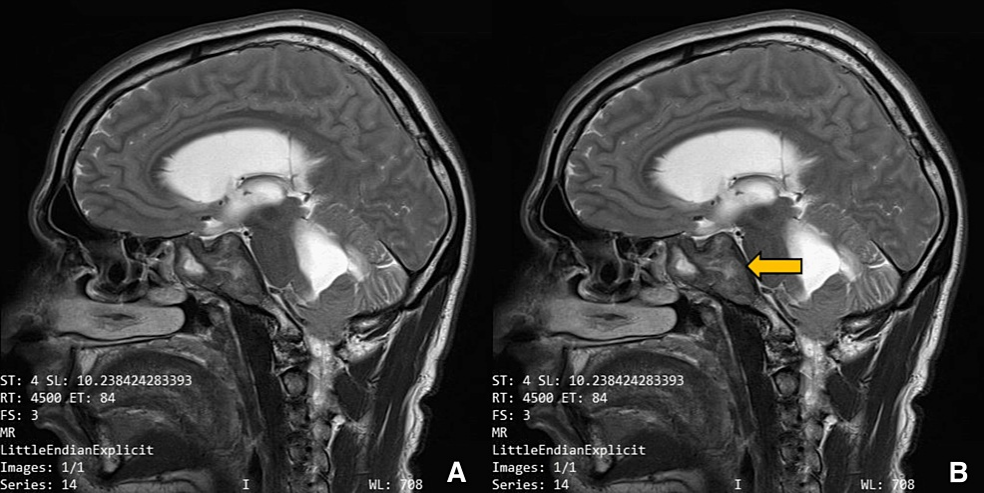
Preoperative imaging for patient 1. Plain T2-weighted sagittal image showing sellar, suprasellar lesion. (A) Without ROI, and (B) with ROI. The yellow arrow points to the lesion which is hypointense surrounded by hyperintense regions which are specific to *Aspergillus* infection.
